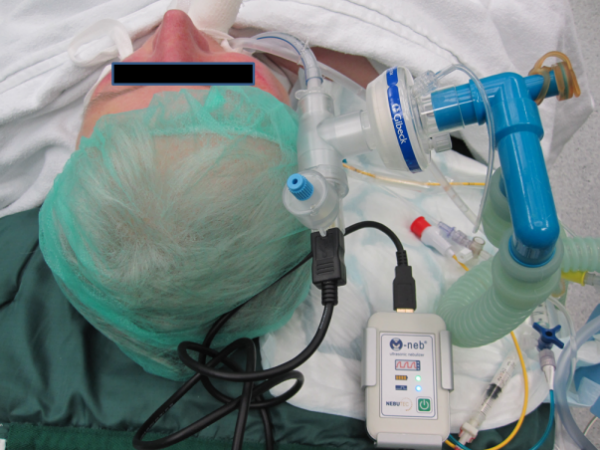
Intraoperative selective pulmonary vasodilation with inhaled iloprost via ultrasonic nebulizer (m-neb^®^, nebutec Elsenfeld, Germany) in the ventilatory circuit
